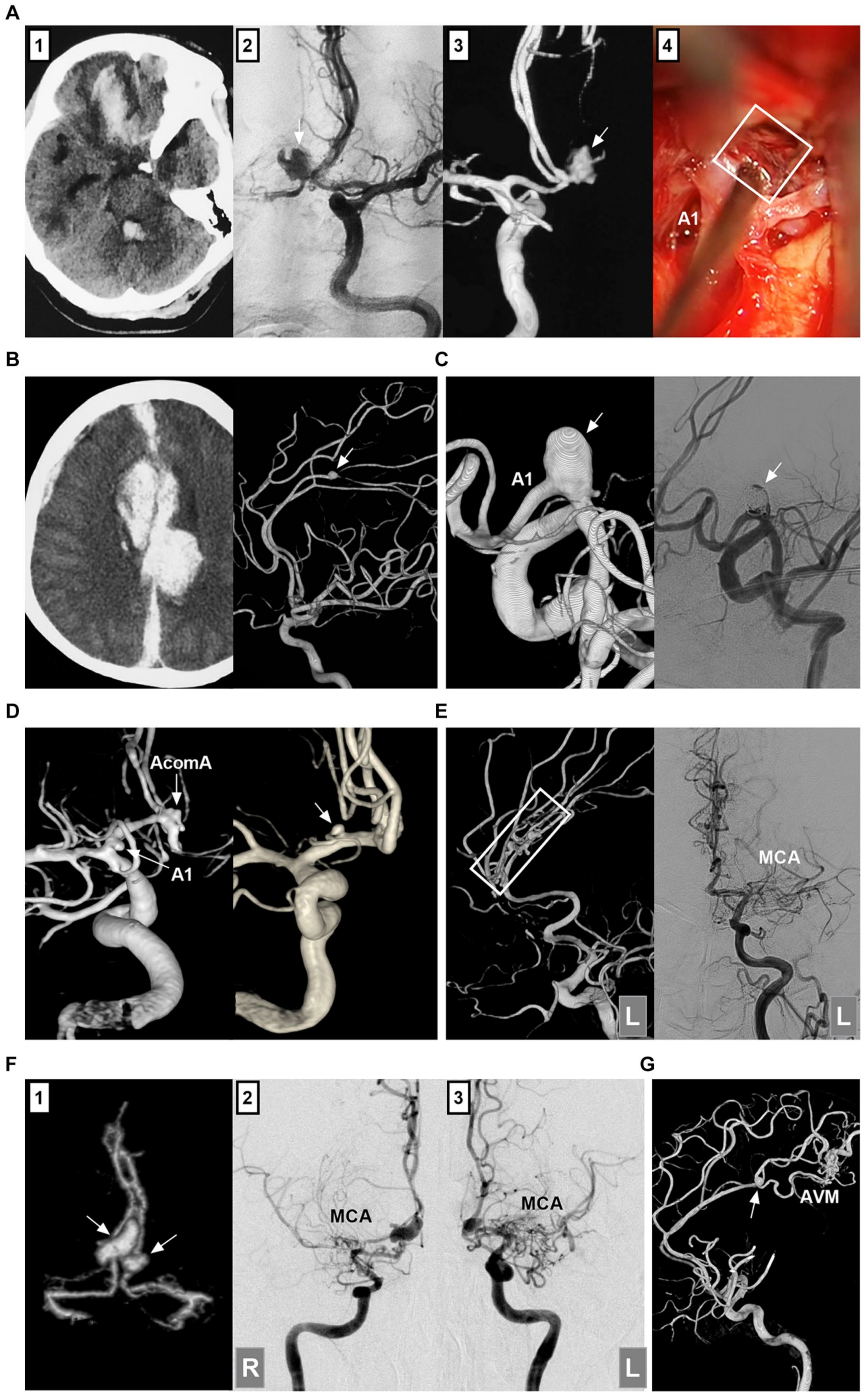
Classifications of ACA aneurysms. (A) Panel 1: CT showing contusion in the frontal lobe and hemorrhage in the fourth ventricle; Panels 2 and 3: DSA showing the traumatic pseudoaneurysm (arrows) at the AcomA; Panel 4: Intraoperative image showing the pseudoaneurysm (frame). (B) Left panel: CT showing traumatic hematoma in the longitudinal fissure and left subdural hematoma. Right panel: 3D-DSA showing the traumatic pseudoaneurysm (arrow) at the distal ACA. (C) Left panel: 3D-DSA showing a dissecting aneurysm in the A1 origin; Right panel: DSA showing the aneurysm after coiling. (D) Left panel: 3D-DSA showing an A1 aneurysm (arrow) and an AcomA aneurysm (arrow); Right panel: 3D-DSA showing an aneurysm (arrow) at the origin of the recurrent artery of Heubner. (E) Left panel: 3D-DSA of the left ICA showing multiple aneurysms (frame) at the distal ACA; Right panel: DSA of left ICA showing the occluded MCA. (F) Panel 1: CTA showing mirror-like distal ACA aneurysms (arrows); Panels 2 and 3: DSA of ICAs showing bilateral twig-like MCAs. (G) 3D-DSA showing an AVM with a flow-related distal ACA aneurysm (arrow). 3D, three-dimensional; A1, first segment; ACA, anterior cerebral artery; AcomA, anterior communicating artery; AVM, arteriovenous malformation; CT, computed tomography; CTA, CT angiography; DSA, digital subtracted angiography; ICA, internal carotid artery; L, left; MCA, middle cerebral artery; R, right.
